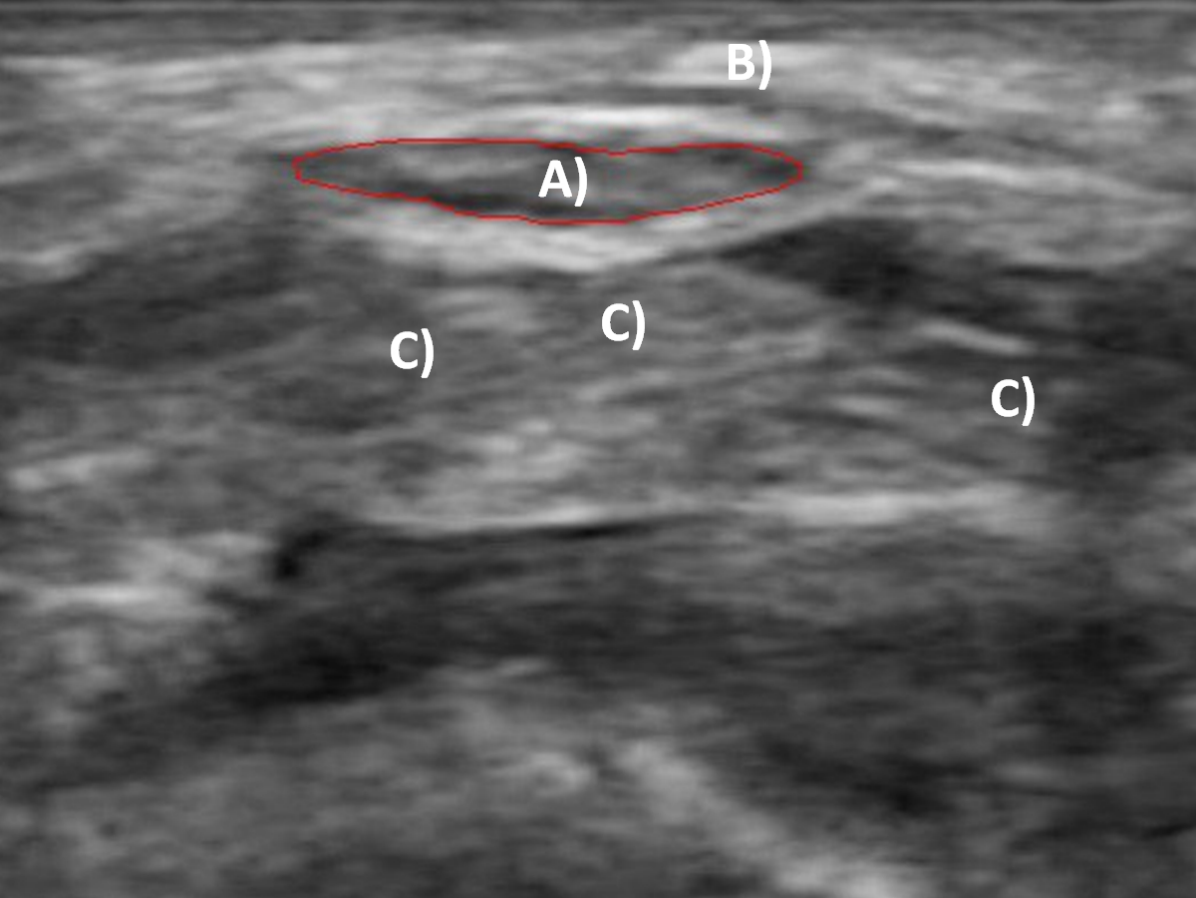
Transverse ultrasound image of the carpal tunnel. (A) Traced median nerve; (B) transverse carpal ligament; (C) flexor tendons.
